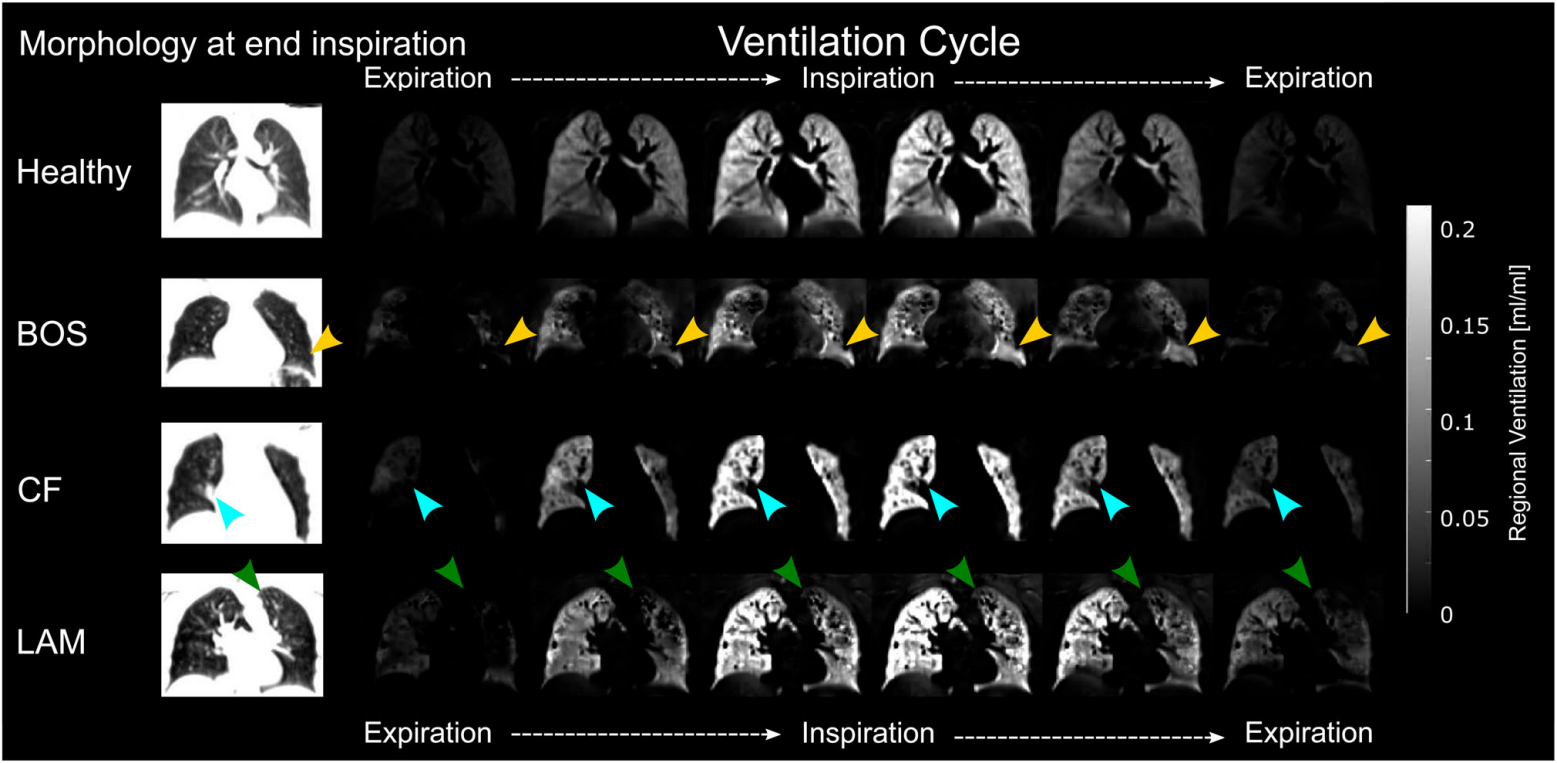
Comparison of three‐dimensional PREFUL (phase‐resolved functional lung) FLORET (fermat‐looped orthogonally encoded trajectories) ultrashort echo time MRI–derived ventilation cycle. Healthy volunteer (*first row*, male, 13 years old), cystic fibrosis (CF; second row, female, 25 years old) patient, bronchiolitis obliterans syndrome (BOS; *third row*, male, 24 years old) patient, and lymphangioleiomyomatosis (LAM; *fourth row*, female, 39 years old) patient are presented. Note homogenous ventilation cycle for the healthy volunteer and no ventilation filling for consolidation in the right lung of the CF patient (*cyan arrows*). For both BOS and LAM subjects, the ventilation dynamics are much more heterogeneous. Dynamic filling asynchronization is indicated with yellow arrows for BOS and green arrows for LAM subjects, suggesting abnormal airflow related to airway narrowing.
